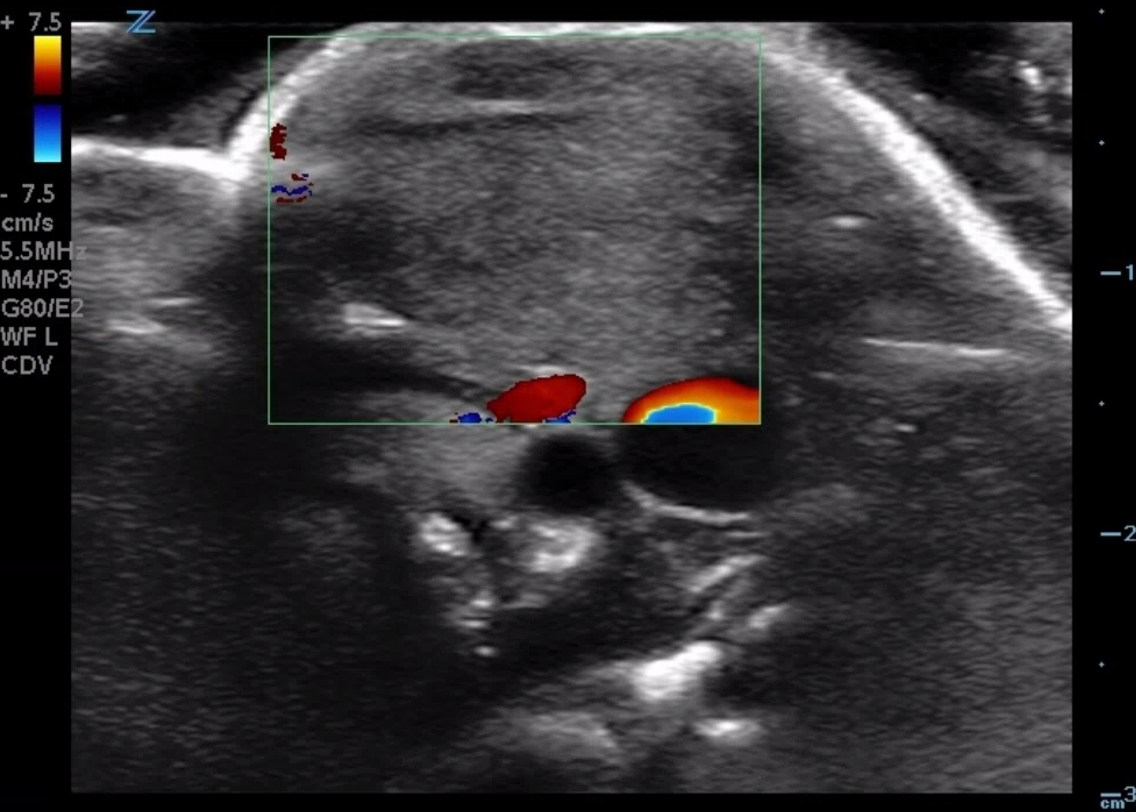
Color Doppler view demonstrates no enhanced vascularity within the mass.
